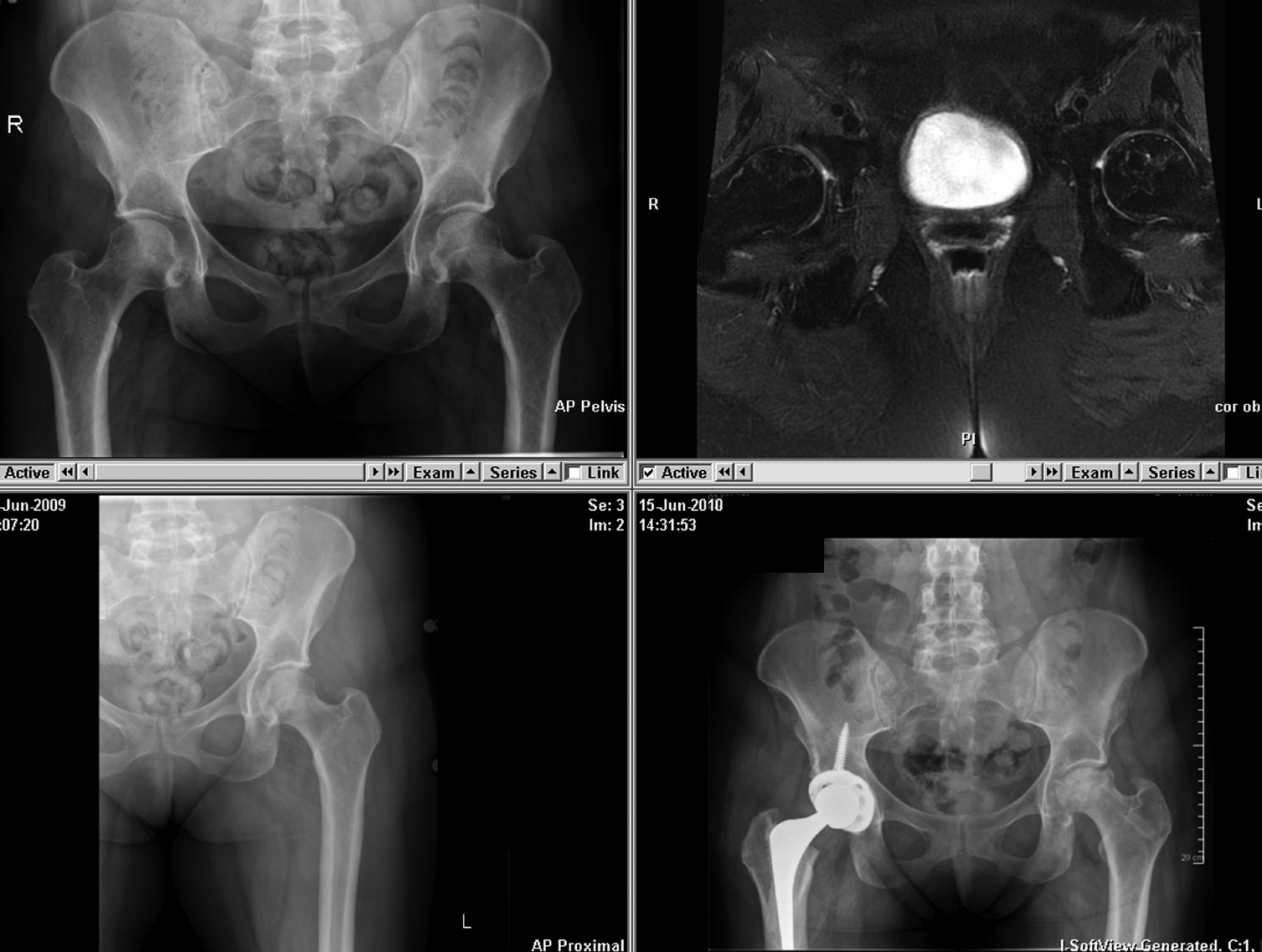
A 43-year-old female on steroids for cancer treatment presents with bilateral hip involvement. Upper left: anteroposterior (AP) pelvis radiograph taken on presentation. Lower left: AP of the left femur taken on presentation. Upper right: T2 coronal oblique MRI showing significant bilateral head involvement prior to treatment. Lower right: AP pelvis shows complete collapse of the left femoral head resulting in total hip arthroplasty 1 year post-operative.
